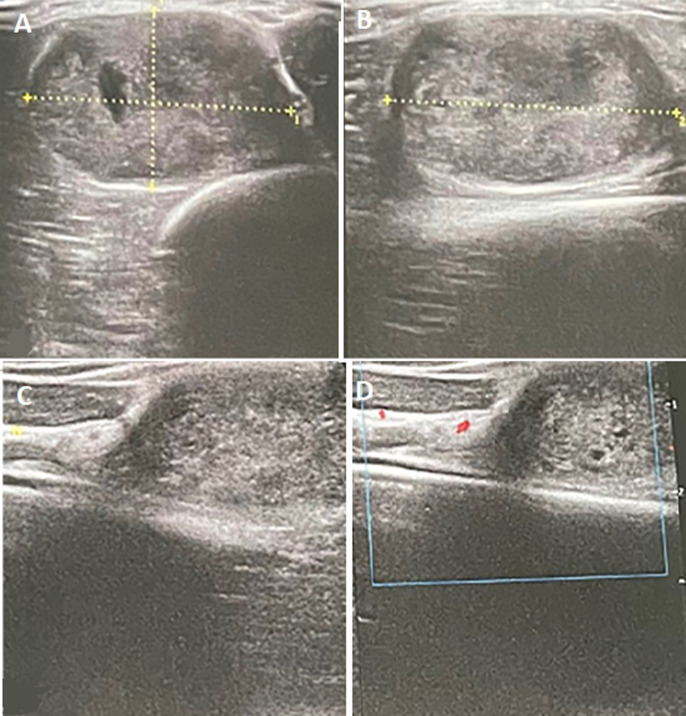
ultrasound aspects; A,B) measures of the mass 27mm/30mm/19mm; (C,D) ultrasound showing a fusiform, homogeneous and well-defined mass in relation to the course of the radial nerve
